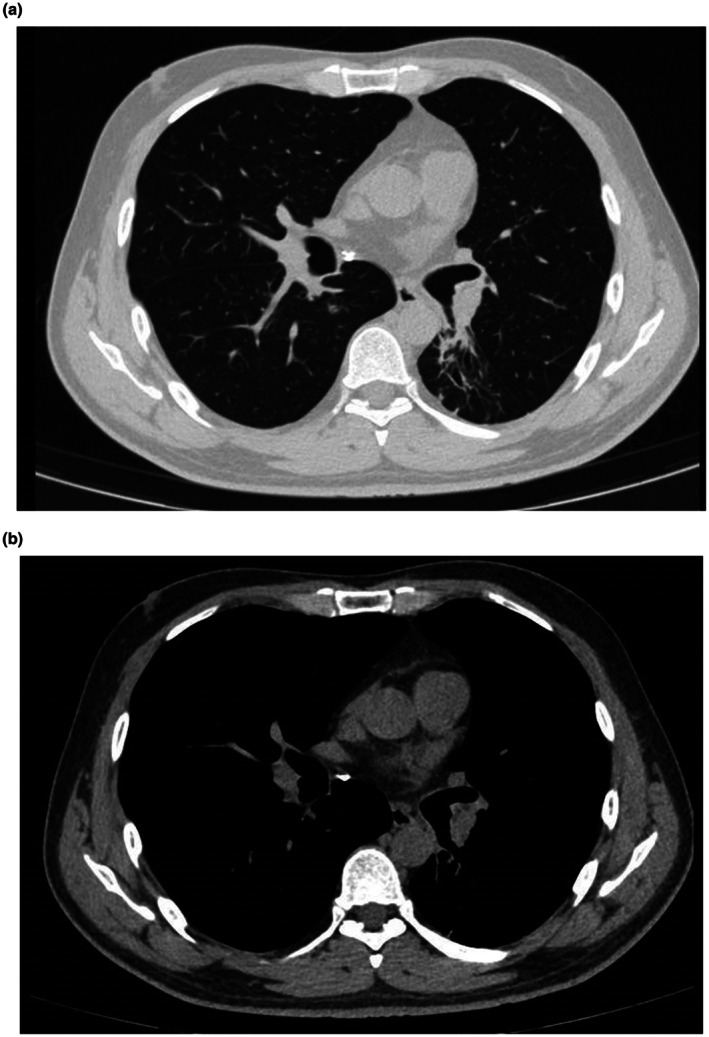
Follow‐up chest CT showing the airways unobstructed and the left lower lung re‐expanded. (a) Lung window. (b) Mediastinal window.
